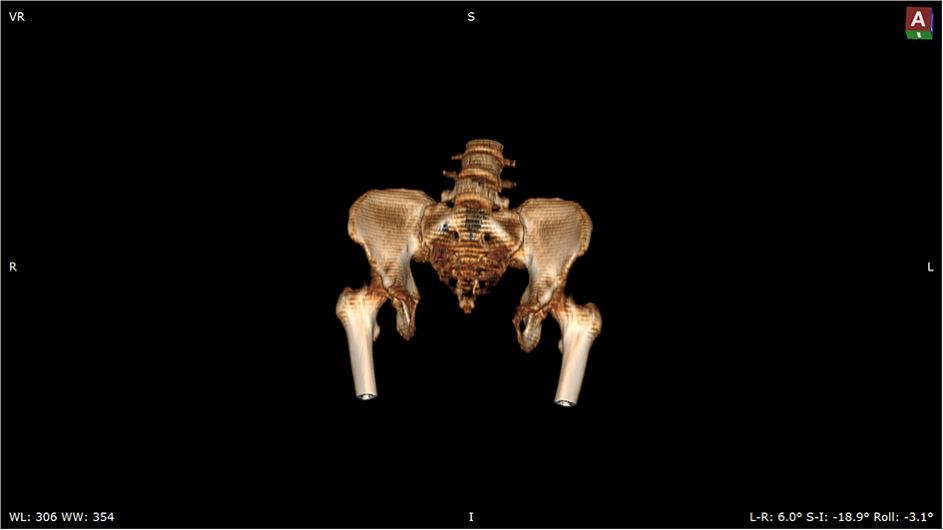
Three dimensional-computed tomography reveals the separated pubic symphysis.
